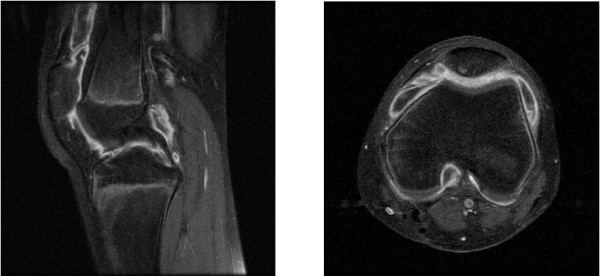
Contrast-enhanced magnetic resonance imaging (fat suppression T1-weighted) at 12 years showed joint effusion and thickening of the synovial membrane.
